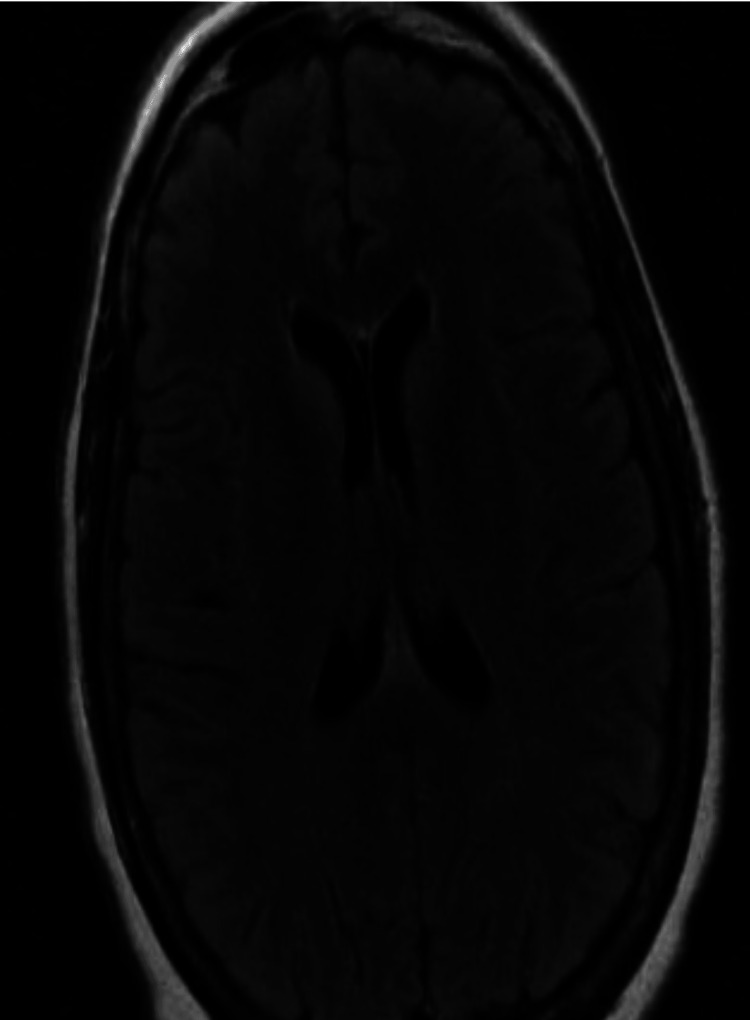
MRI of the brain (without contrast) MRI Brain did not reveal demyelinating plaques or ischemic changes.
